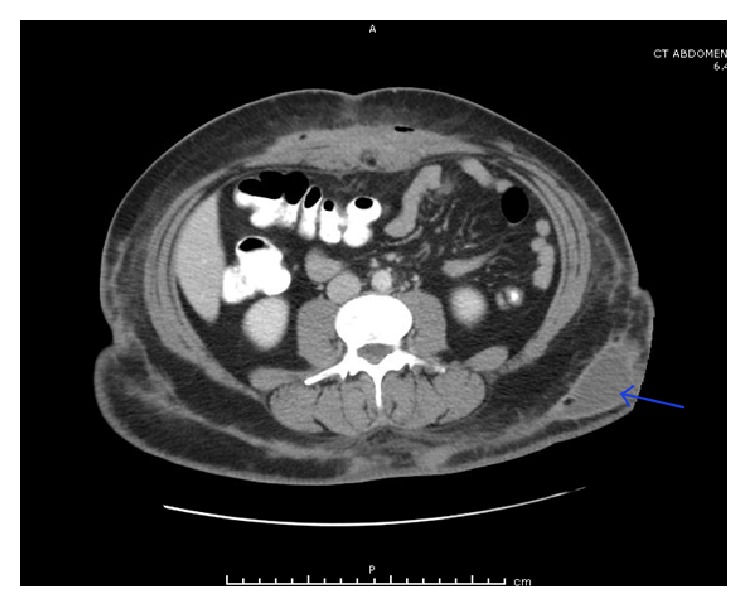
CT abdomen and pelvis with intravenous contrast showing subcutaneous rim-enhancing lesion in the left flank (blue arrow) suggestive of an abscess.
